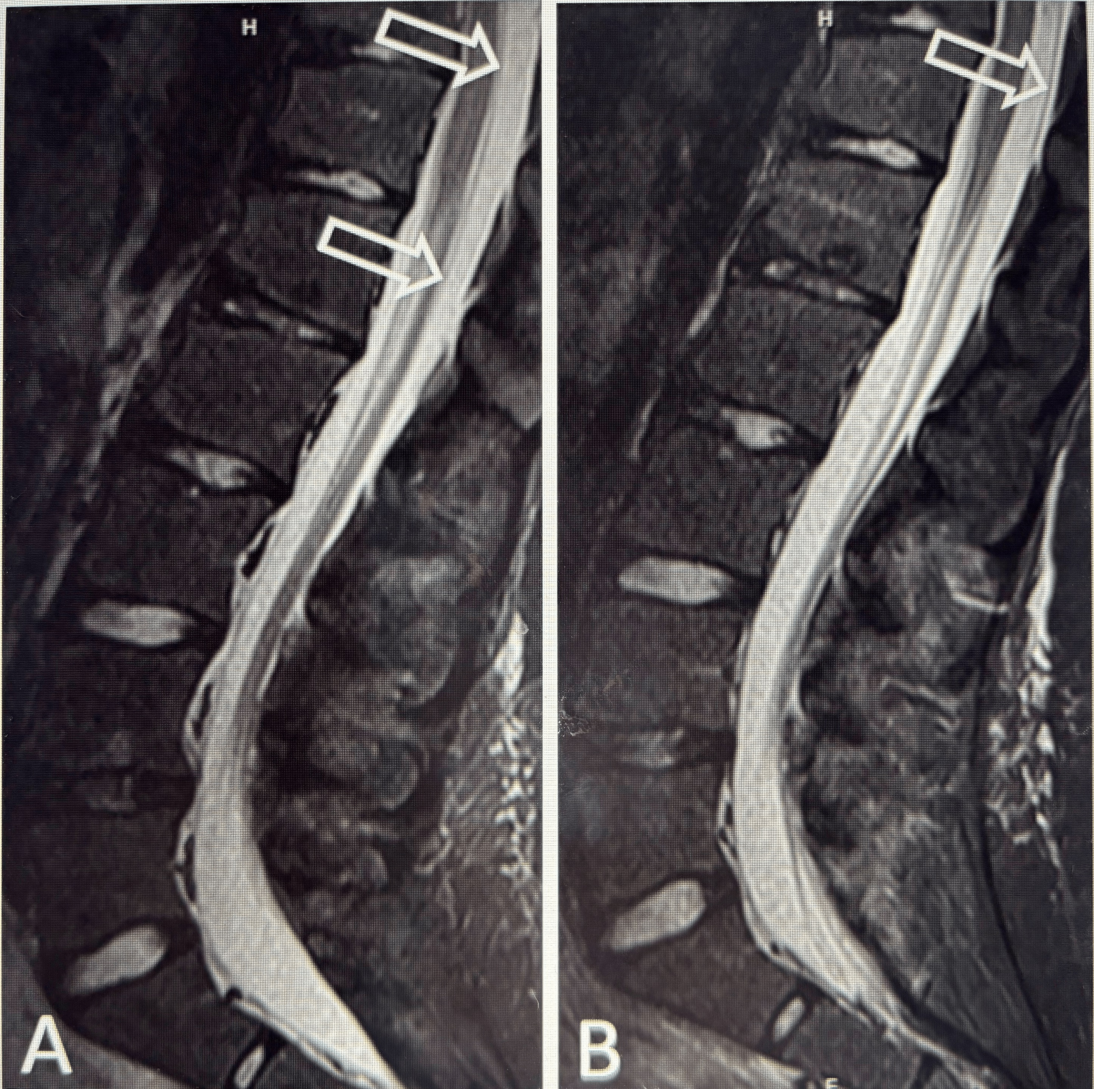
Lumbar spine MRI (Patient 1) Epidural fluid collection on the lumbar spine MRI of Patient 1, which nearly completely resolved after EBP. The sagittal STIR image of the lumbar spine four days before the EBP shows a large fluid collection posterior to the dura (A, open arrows), which significantly decreased in the subsequent MRI five days after the EBP (B, open arrow). Note the position of the dura, which is displaced anteriorly close to the conus medullaris in the pre-EBP MRI versus the normal posterior positioning close to the ligamentum flavum in the post-EBP MRI. EBP: epidural blood patch; STIR: short tau inversion recovery
